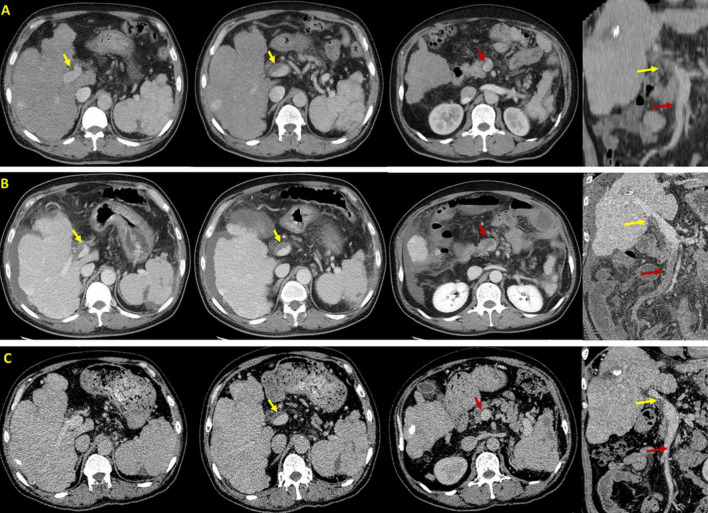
Axial and coronal contrast-enhanced computed tomography scans in this patient. (A) Before EVT, CT scans performed on 7 November 2020 demonstrated PVT (yellow arrow) without SMV thrombosis (red arrow). (B) After EVT, CT scans performed on 29 November 2020 demonstrated PVT (yellow arrow) with acute SMV thrombosis (red arrow). (C) After anticoagulation, CT scans performed on 19 January 2021 demonstrated that PVT remains stable (yellow arrow) and SMV thrombosis disappeared (red arrow).
